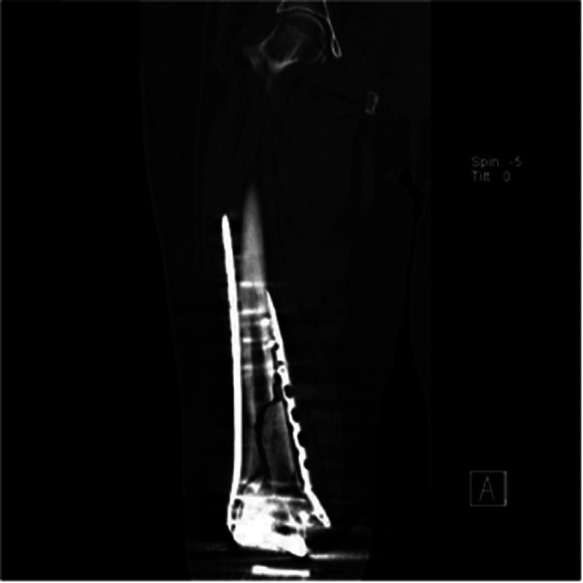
Coronal CT image of the right femur one year after surgery.
